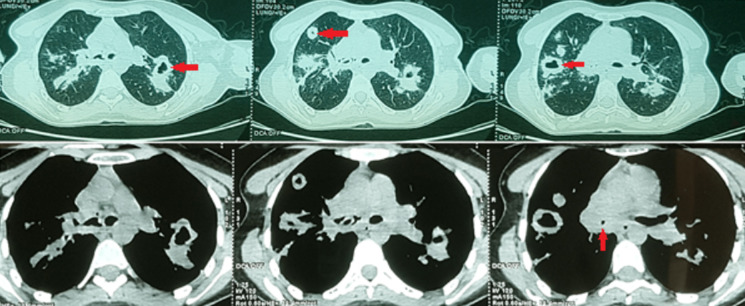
CT scan with multiple bilateral excavated nodules, reduction in the right main bronchus caliber with mediastinal lymphadenopathy
